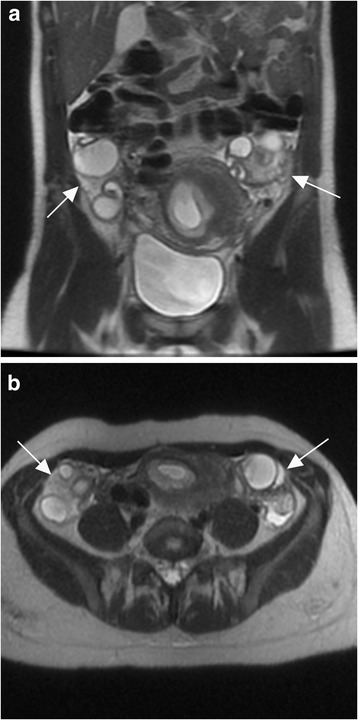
Stimulated ovaries without torsion. Coronal (a) and axial (b) T2-weighted MR images of a 37-year-old woman with right lower quadrant pain at 8 weeks gestational age demonstrate symmetrically enlarged ovaries (arrows) with increased T2 signal intensity of the ovarian stroma and multiple large follicles. The patient had a known diagnosis of ovarian hyperstimulation syndrome requiring paracenteses, was observed clinically, and was discharged without surgery
